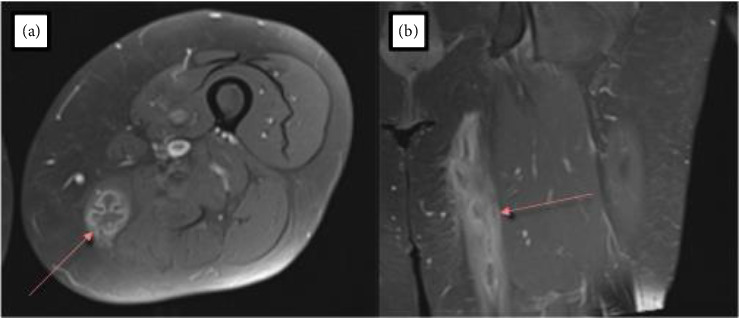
(a) Axial postcontrast T1-weighted MRI demonstrating abscess formation in the gracilis and (b) coronal view showing longitudinal gracilis involvement.
